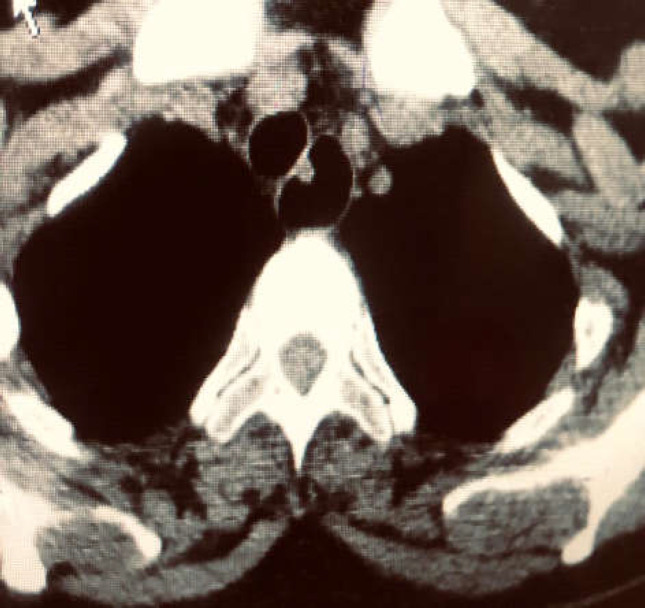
A mediastinal CT scan showed a non-enhancing lesion with well-defined margins in the middle to distal esophagus with no invasion or lymph node involvement
